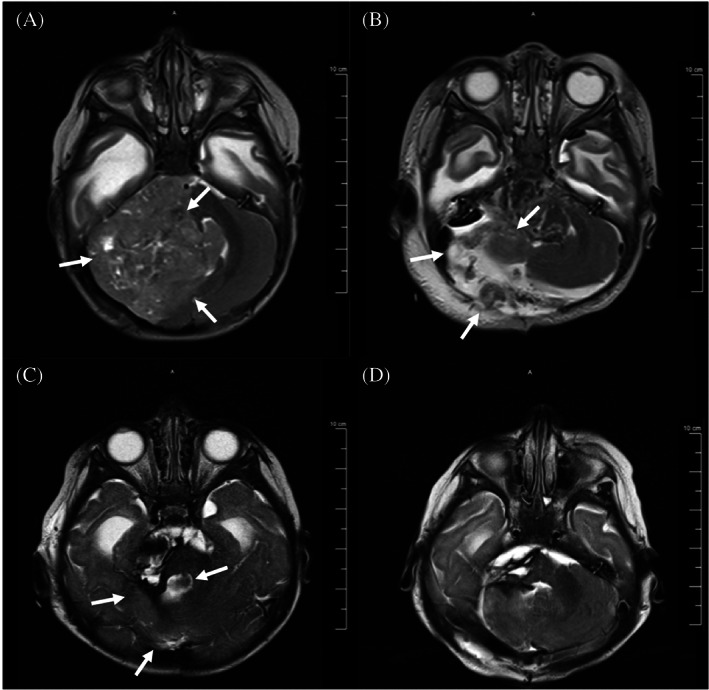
Axial T2 MRI, here depicting the level of the posterior fossa: (A) tumor at diagnosis, 6.5 × 6.5 × 4.4 cm (margins portrayed by white arrowheads) (December 2016); (B) residual tumor following the initial surgical resection, 4.9 × 4.2 × 4.3 cm (margins indicated by white arrowheads) (December 2016); (C) residual tumor following the fourth cycle of chemotherapy, 4.5 × 2.4 × 2.3 cm (margins indicated by white arrowheads) (May 2017); and (D) following gross total resection of residual tumor (June 2017).
